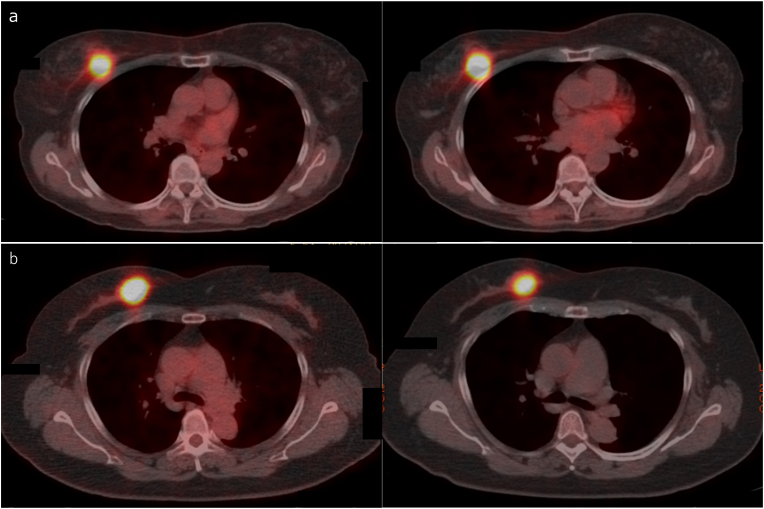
Representative images of FDG PET/CT at early (left) and delayed (right) phases. (a) A 67-year-old woman with infiltrating duct carcinoma not otherwise specified, T2N0M0 stage IIA, luminal B-like subtype, nuclear grade 2, SUVmax1 10.2, SUVmax2 15.6, RI 52.9% in right breast. The patient experienced recurrence of bone metastasis 29 months after surgery and died 60 months after surgery. (b) A 63-year-old woman with infiltrating duct carcinoma not otherwise specified, T2N0M0 stage IIA, luminal B-like subtype, nuclear grade 2, SUVmax1 16.8, SUVmax2 17.2, RI 2.4% in right breast. The patient remained relapse-free 98 months after surgery. RI, retention index; SUVmax, maximum standardized uptake value.
